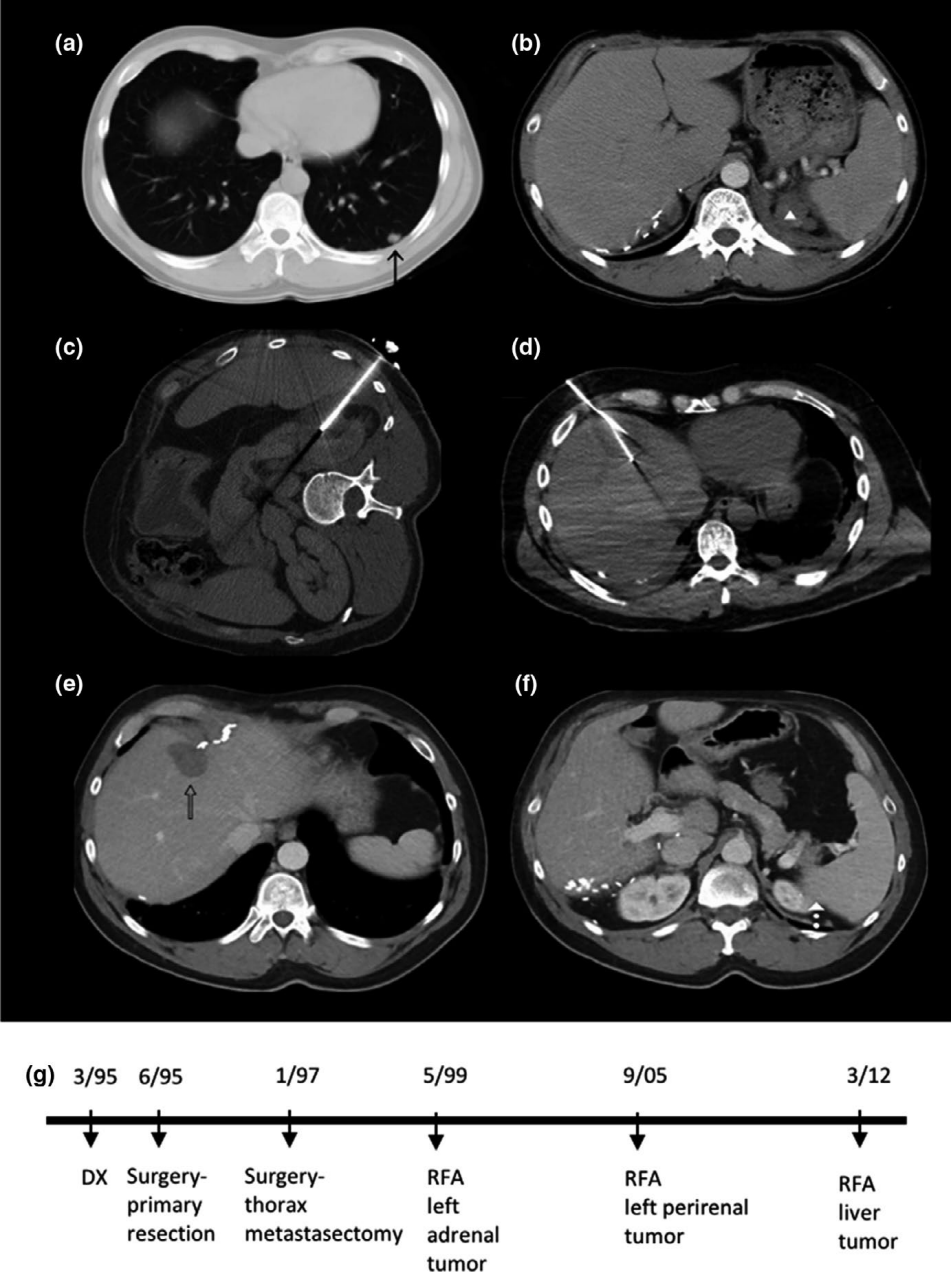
Case example. CT images of a patient that was diagnosed with stage IV adrenocortical carcinoma (ACC) and received his first image‐guided locoregional therapy (IGLT) 50 months after diagnosis. Contrast enhanced axial CT images pre‐ablation show lung metastasis (A, arrow) and left adrenal bed tumors (B, arrowhead). The patient was also treated with mitotane, systemic chemotherapy, and thoracic metastectomy. Three RFA sessions were performed at the left adrenal bed, left perirenal region, and segment 8 in the liver (intra‐procedural non‐contrast CT C, D) over a time span of 154 months. Long term surveillance with contrast enhanced axial CT 38 months following the last treatment session shows completely treated tumors (E, F). Timeline of interventions is provided in (G). This patient is alive 292 months following his initial diagnosis with no imageable active disease
